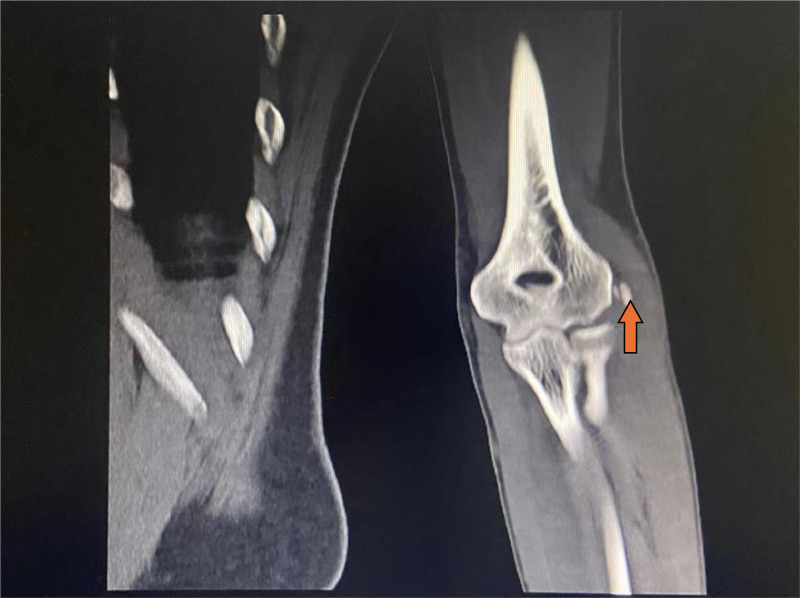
A radiograph of the right-hand shows calcification of the lateral epicondyle.
